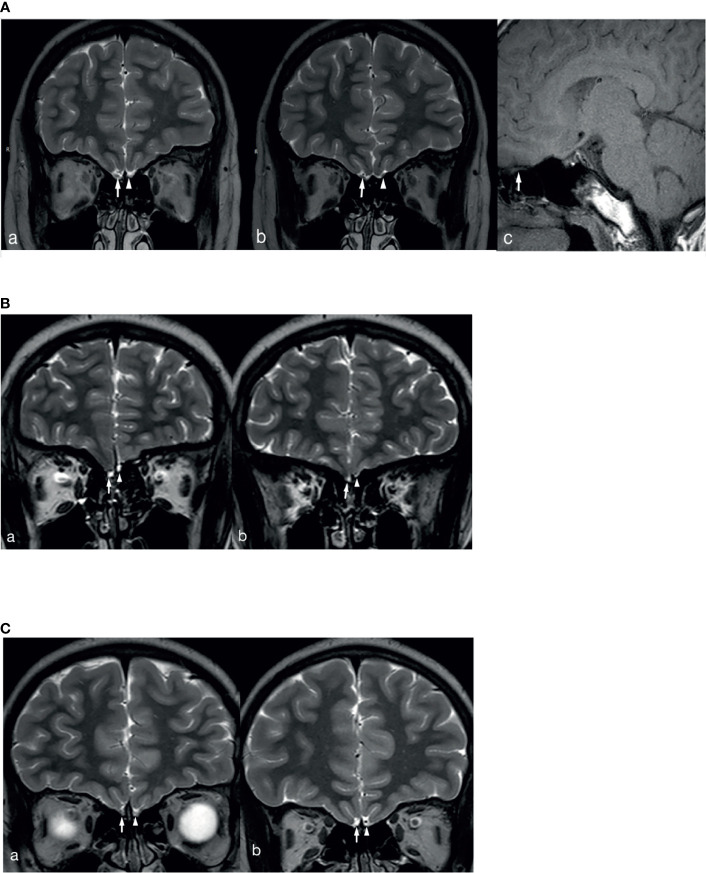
MRI images of 3 patients with KS. (A) The right olfactory bulb and olfactory tract of the patient are normally displayed (White long arrows in a and b show the right olfactory bulb and olfactory tract, white long arrow c shows sagittal position of right olfactory tract), the left olfactory bulb is not displayed (White short arrow in a), and the left olfactory tract (White short arrow in b) is smaller than the opposite side. (B) The bilateral olfactory bulbs and olfactory tracts of the patient are not clearly displayed (White long arrows in a and b show the right olfactory bulb and olfactory tract, white short arrows in a and b show the left olfactory bulb and olfactory tract), and the left olfactory sulcus is shallower than the opposite side (a and b). (C) The bilateral olfactory bulbs and olfactory tracts of the patient are not clearly displayed (White long arrows in a and b show the right olfactory bulb and olfactory tract, white short arrows in a and b show the left olfactory bulb and olfactory tract), and the bilateral olfactory sulcus is shallow (a and b).
